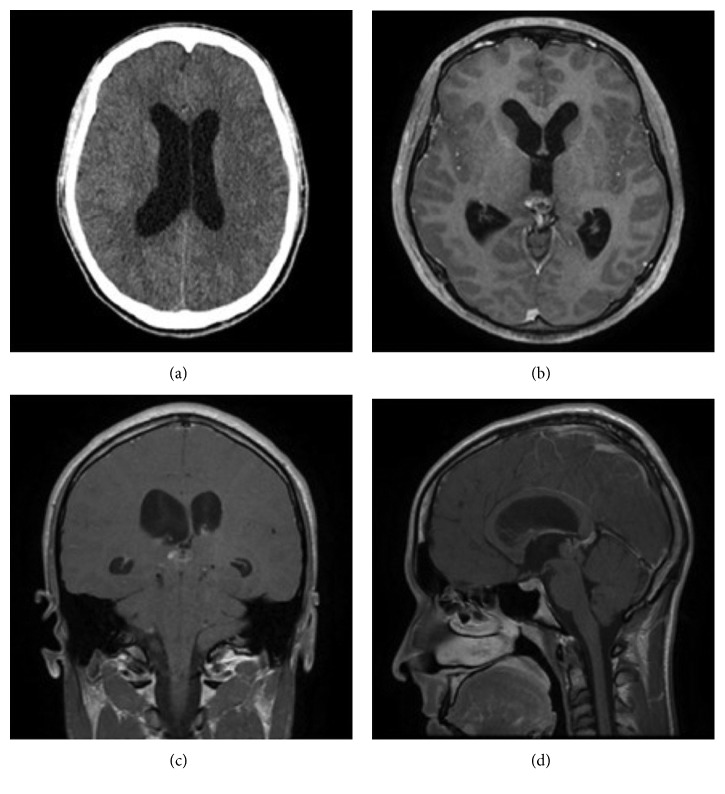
CT scan and MRI at admission documented acute obstructive hydrocephalus caused by a pineal region mass.
